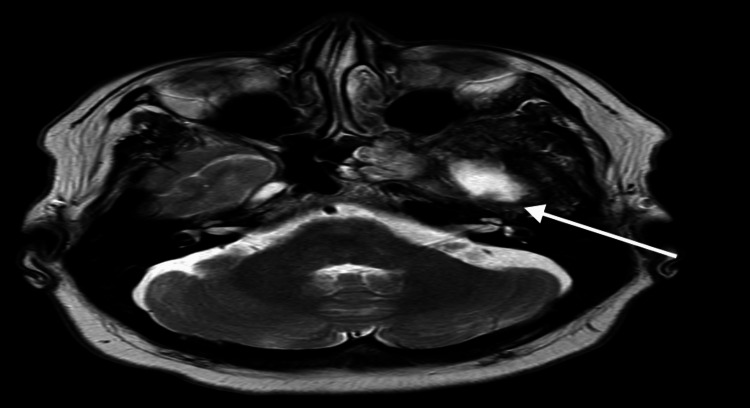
Diffusion-weighted imaging (DWI) MRI showing encephalomalacia in the left temporal lobe (white arrow). The annotated area highlights postoperative structural change without evidence of recurrence or mass effect.
